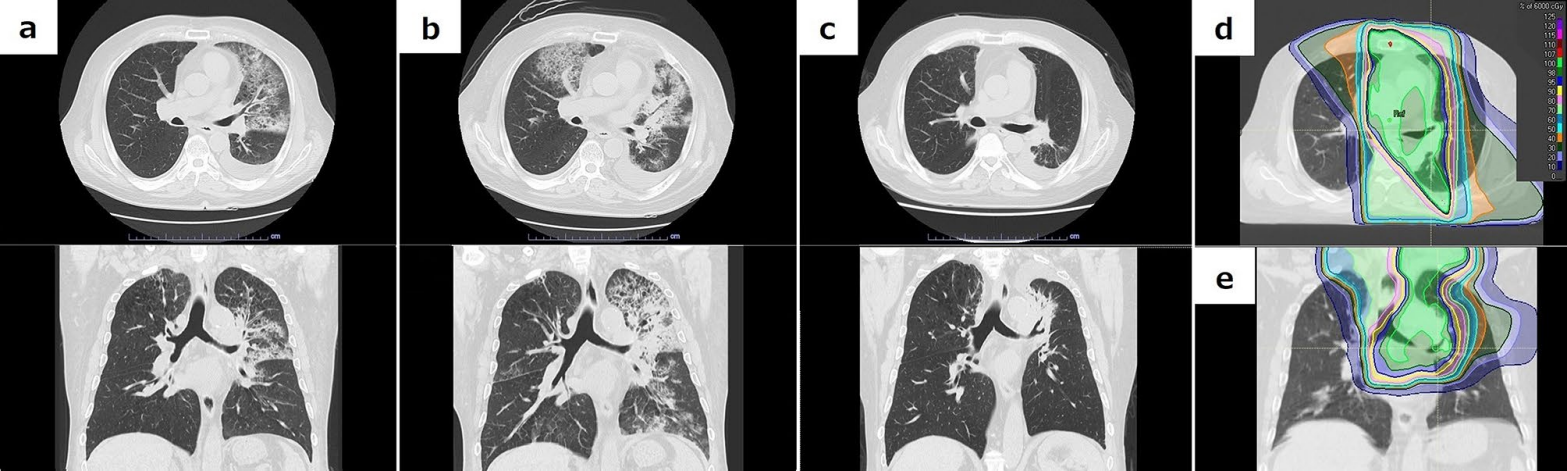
Axial (upper) and coronal (lower) computed tomography (CT) images of a representative patient Interstitial lung disease/radiation pneumonitis (ILD/RP) arising from the low-dose irradiated area after chemoradiotherapy (CRT) in patient #10 in Table 3. (a): On the 37th day after the completion of CRT, the patient had received 3 cycles of durvalumab before discontinuing due to diagnosis of Grade 2 ILD/RP. The shadow disappeared soon after initiation of prednisone (PSL, 80 mg/day = 1 mg/kg/day). (b): On the 89th day, when PSL was being tapered to 20 mg/day, infiltrative shadows and ground-glass opacities reappeared and spread over the irradiated area with worsened dyspnea, leading to diagnosis of Grade 3 ILD. (c): On the 173rd day, the infiltrative shadow and ground-glass opacities disappeared after increasing the dose of PSL, but fibrosis appeared limited to the high-dose irradiated areas. (d): axial and (e): coronal CT images show the radiotherapy dose distribution in this patient
